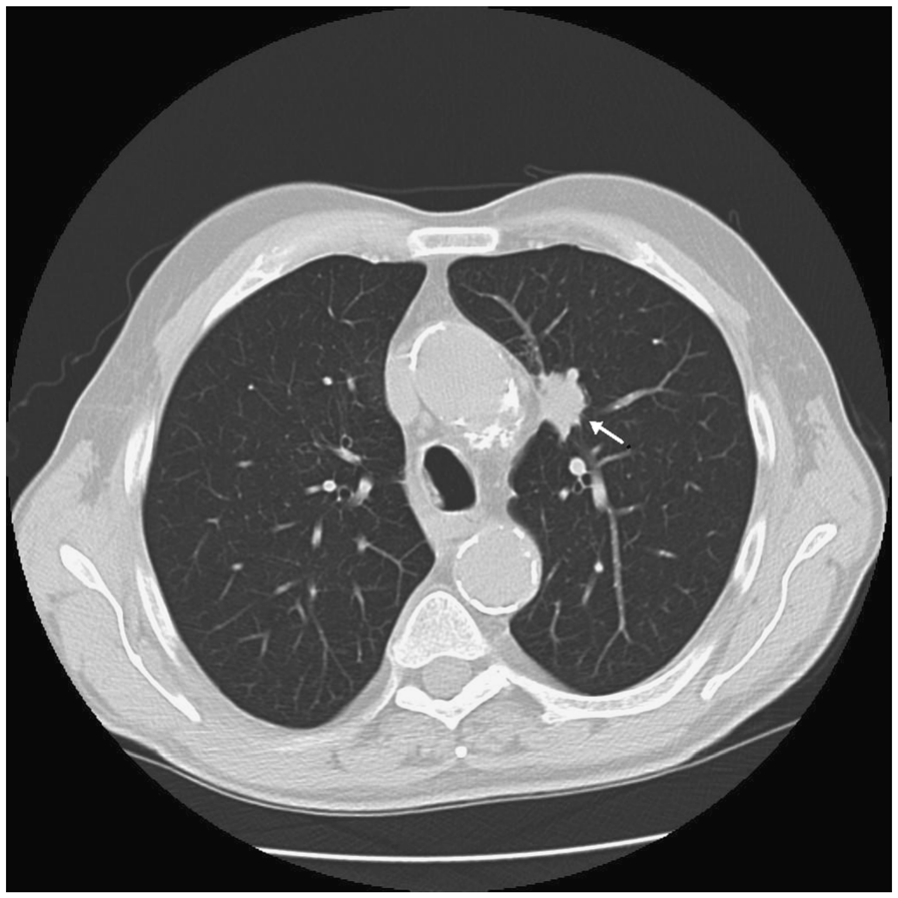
Chest computed tomography scan revealing a mass in the superior left lobe (arrow).
